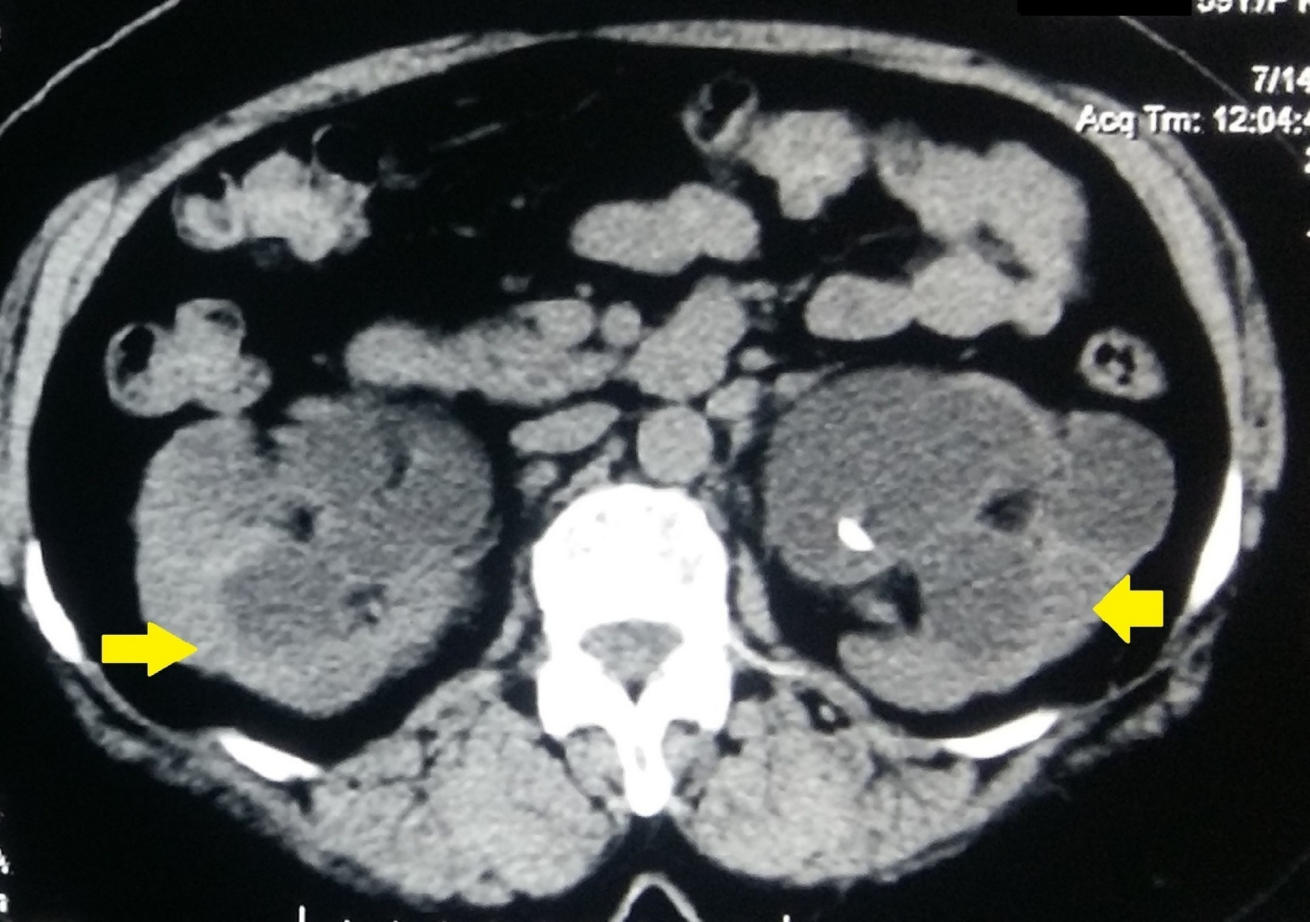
Noncontrast computed tomography showing bilateral gross hydroureteronephrosis and renal cortical thinning (yellow arrows)
